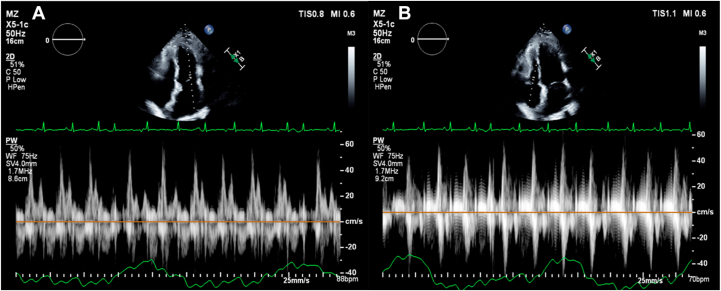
Case 3. Two-dimensional TTE, apical 4-chamber view (A, B) demonstrates mitral and tricuspid inflow variations (both 24%) that did not meet the traditional CP criteria.
